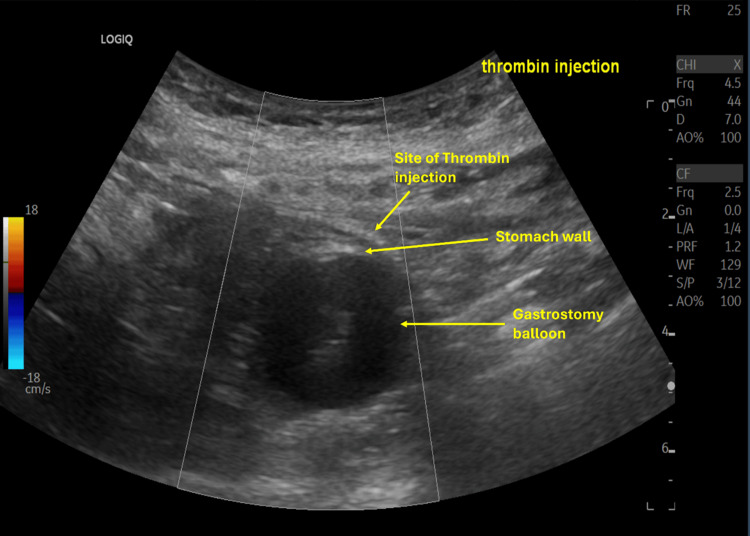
Bedside ultrasound-guided thrombin injection at the gastrostomy site demonstrating hemostasis.
